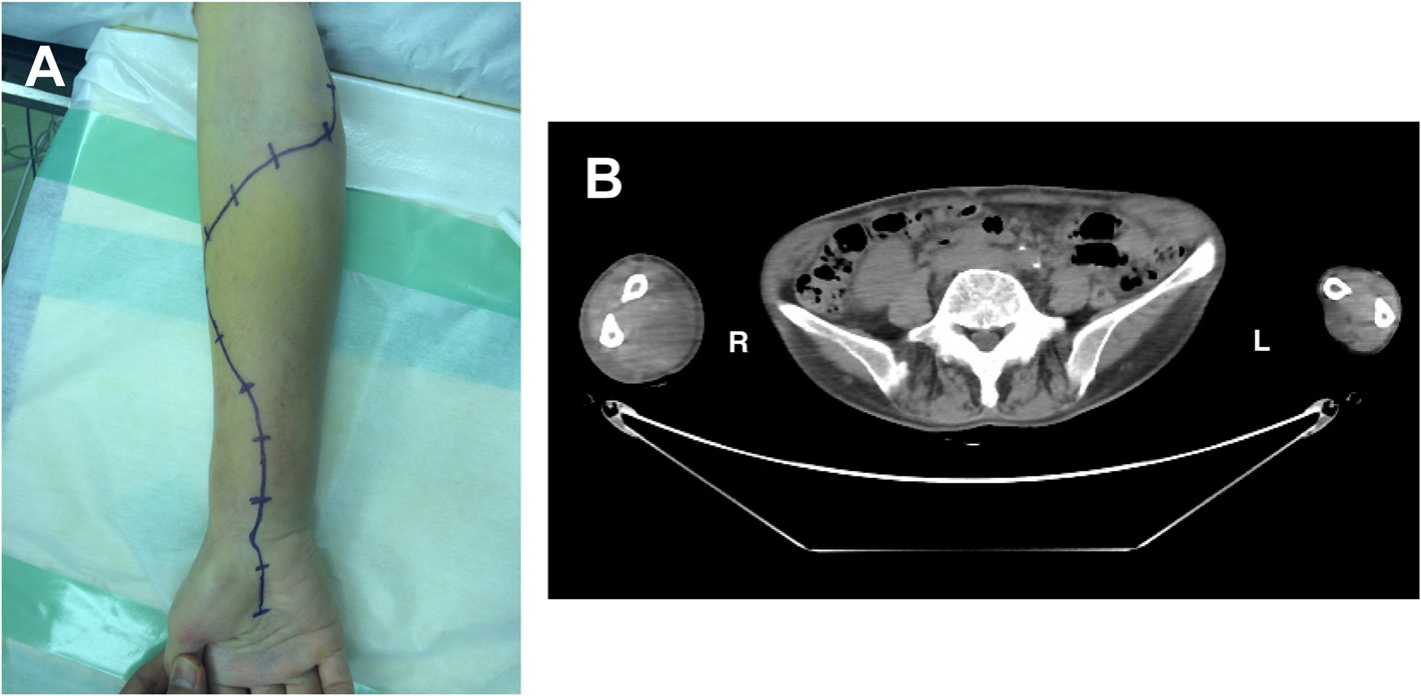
a Swollen right forearm of the patient. b Computed tomography revealing marked swelling of the right forearm compared with the left forearm
